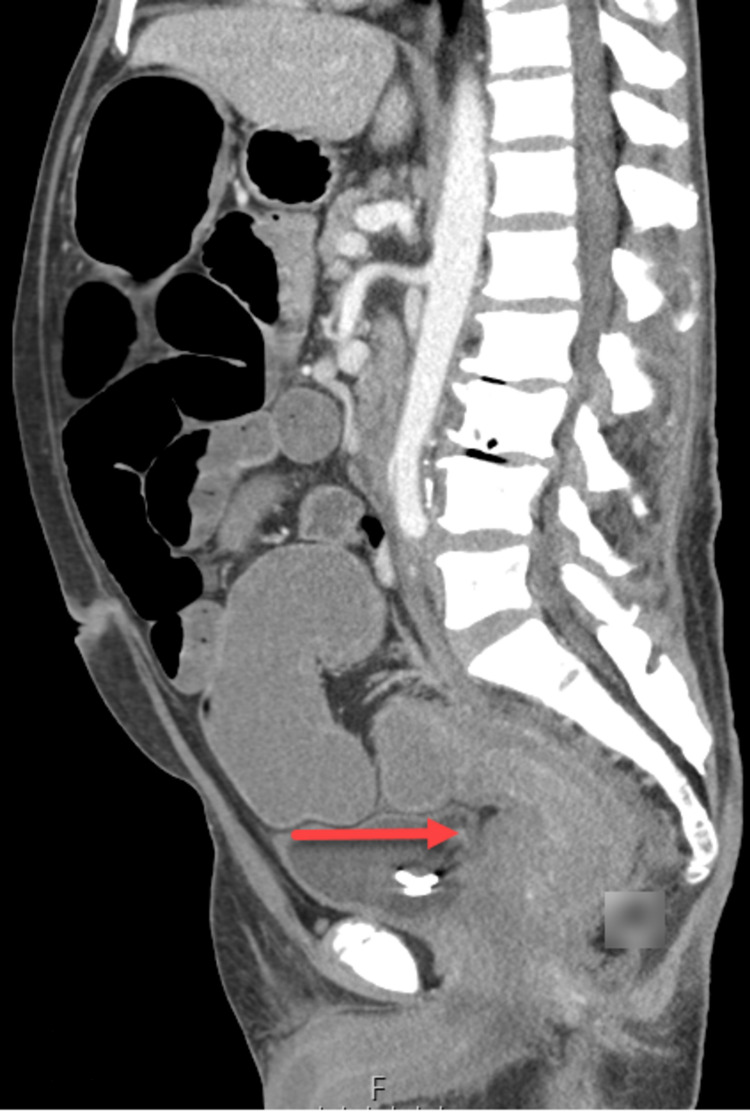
CT sagittal view: red arrow demonstrates infiltrative soft tissue leading to large bowel and rectum obstruction.
